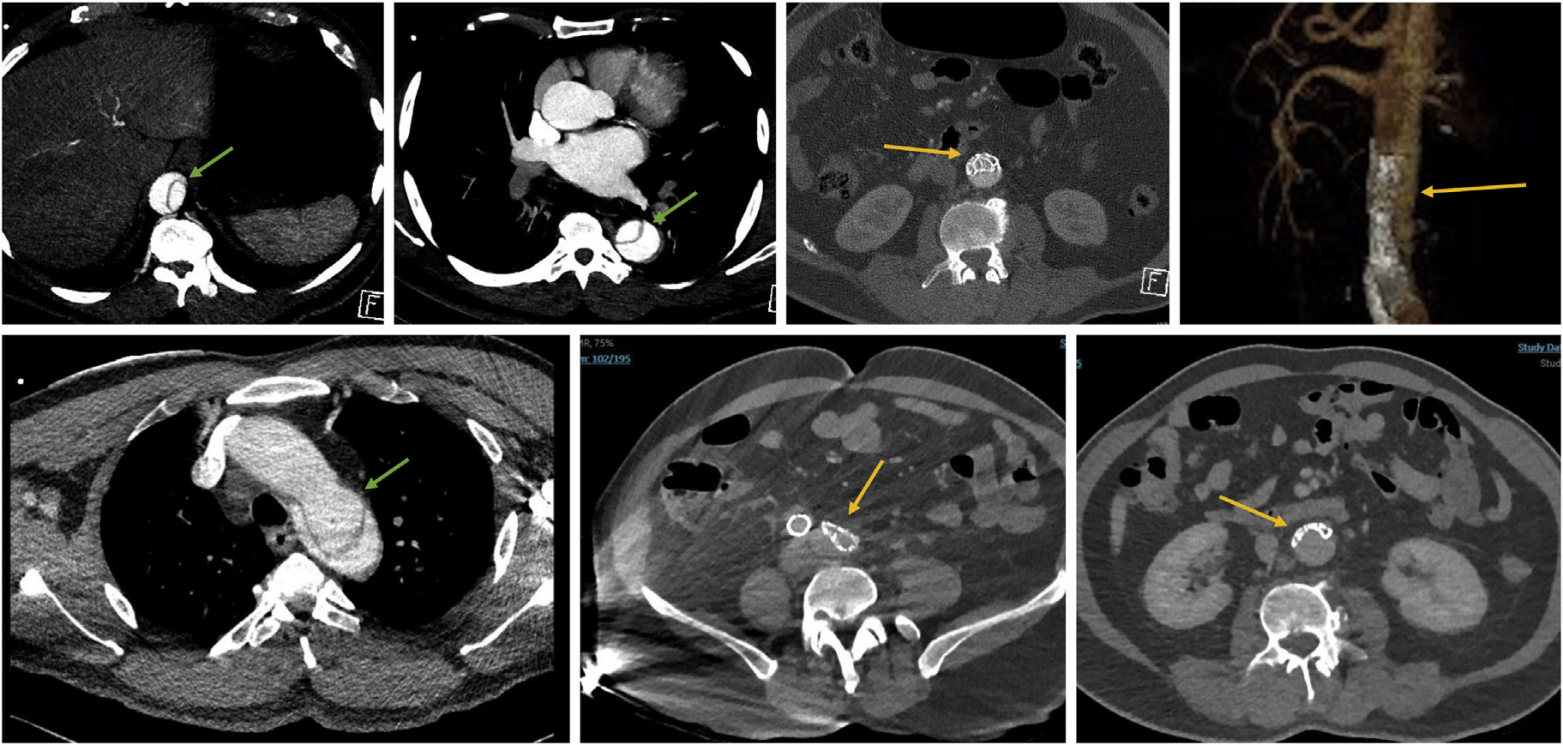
Preoperative computed tomography angiography reveals a type B acute aortic dissection (TBAD) and collapsed infrarenal endograft. Yellow arrows indicate the collapsed infrarenal endograft. *Green arrows* indicate the TBAD, beginning at the level of the left common carotid artery.
